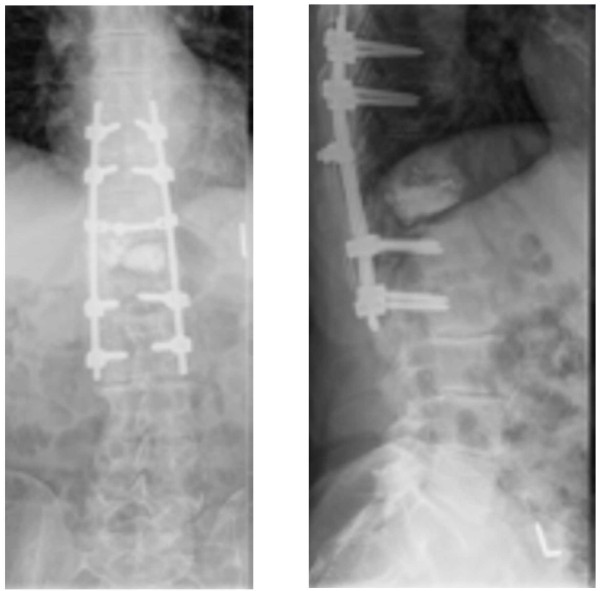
Post-operative X-rays of the patient’s lower thoracic and lumbar spine (anteroposterior and lateral views) showing posterior fixation.
